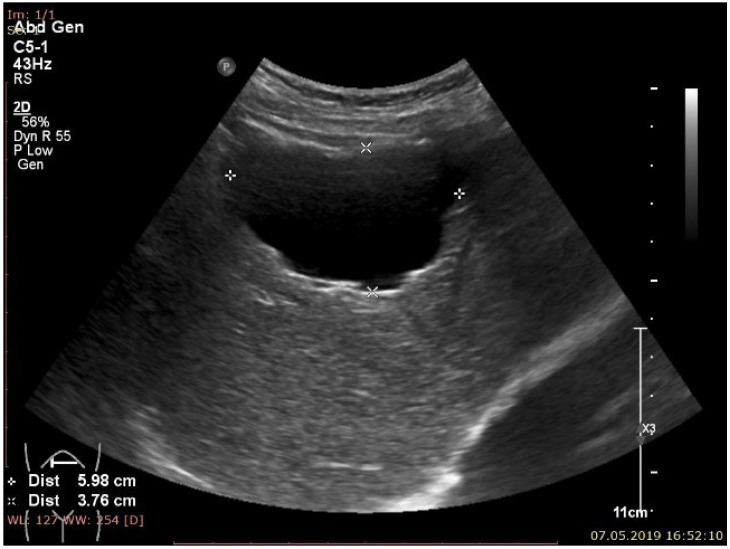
A 35-year-old male presents for consultation for nausea and diarrhea with acute onset. Ultrasound revealed a large, anechoic lesion (between markers x and +) with thin, irregular walls, situated in segment 4–5—typical aspect of simple biliary cyst.
